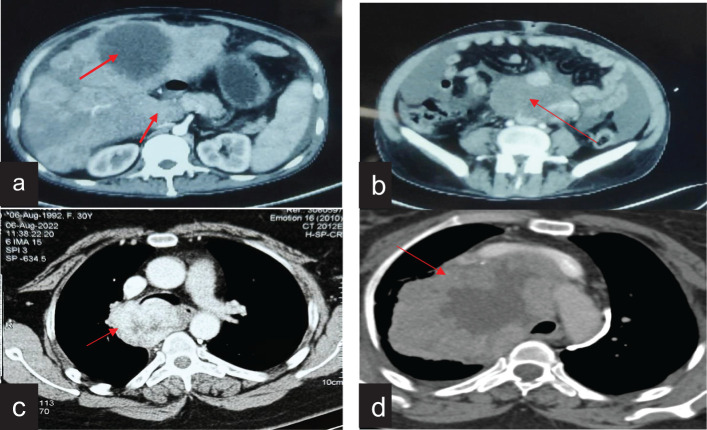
Radiological features of FDCS cases on contrast-enhanced computed tomography. (a) CT of the abdomen showing retroperitoneal FDCS with liver metastasis. (b) CT of the abdomen showing a large retroperitoneal FDCS. (c) CT of the chest showing mediastenial FDCS extending from the lower paratracheal region and abutting the trachea and esophagus. (d) CT of the chest showing a bulky mediastinal FDCS causing bronchial compression and SVC syndrome.
